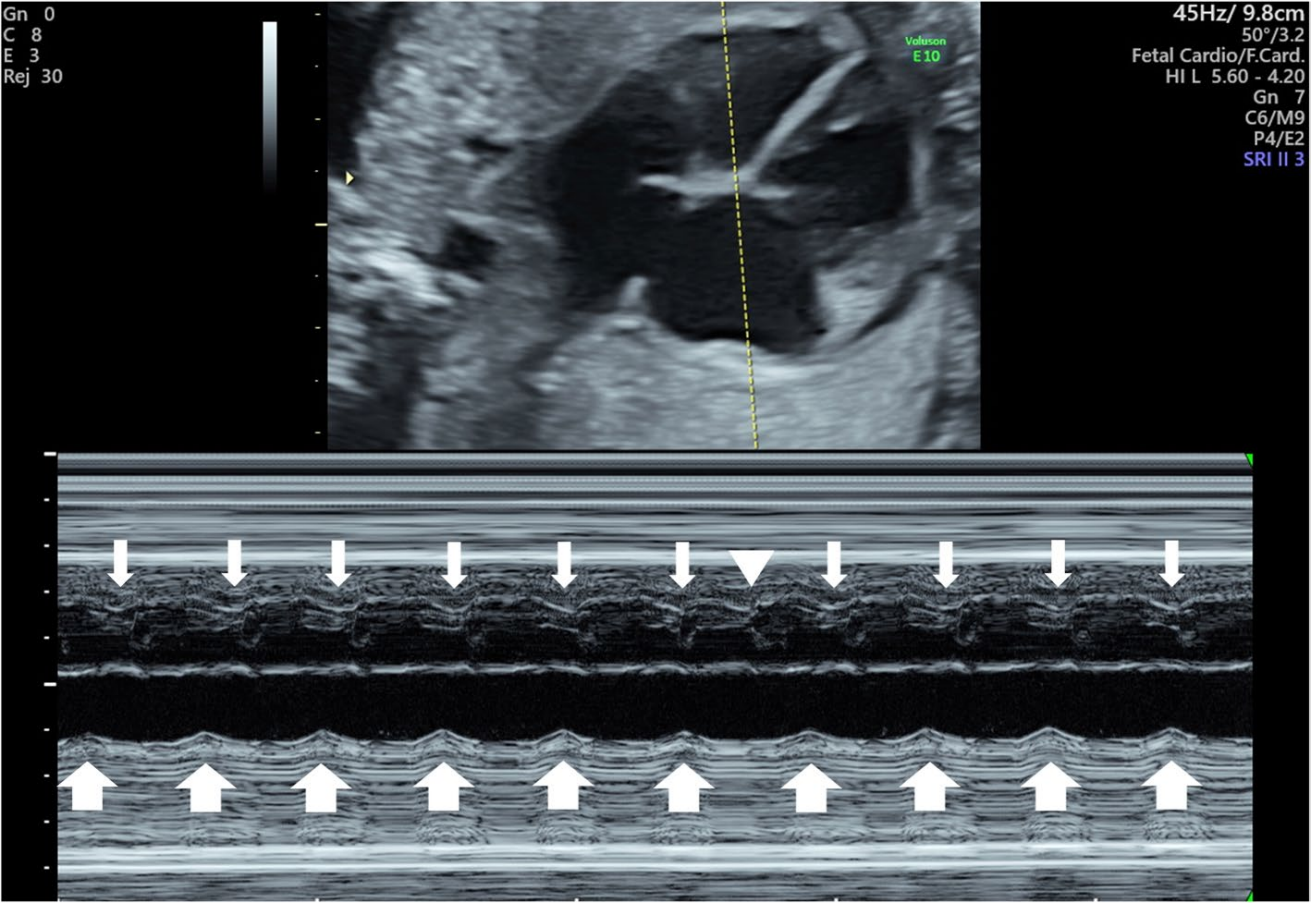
Fetal M-mode ultrasound. Ventriculo-atrial dissociation in atypical junctional ectopic tachycardia; junctional ectopic tachycardia was diagnosed postnatally, and the findings were detected retrospectively. In the four-chamber view of the heart, which shows the left ventricle and right atrium, the wide arrow indicates atrial contraction, the narrow arrow indicates ventricular contraction, and the arrowhead indicates a premature ventricular contraction
